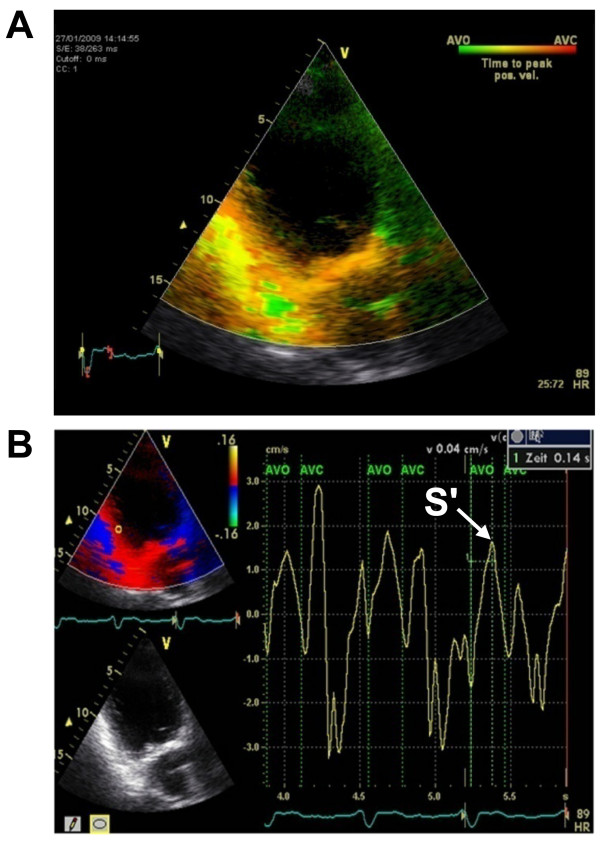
Example of a patient with a reduced acoustic window. A) TSI in the apical two-chamber view. The inferior segment is color-coded in orange. Therefore, S' must be within the middle third of the transaortal flow. This information serves as an intrinsic plausibility control and helps to identify the correct S' in the subsequent TDI measurements - especially under impaired ultrasound conditions as depicted in here. B) TDI in the apical two-chamber view. The interval between the aortic valve opening (AVO) and the peak systolic velocity (S') is 140 ms. In agreement with the TSI data, S' can be found within the middle third of the transaortal flow.
